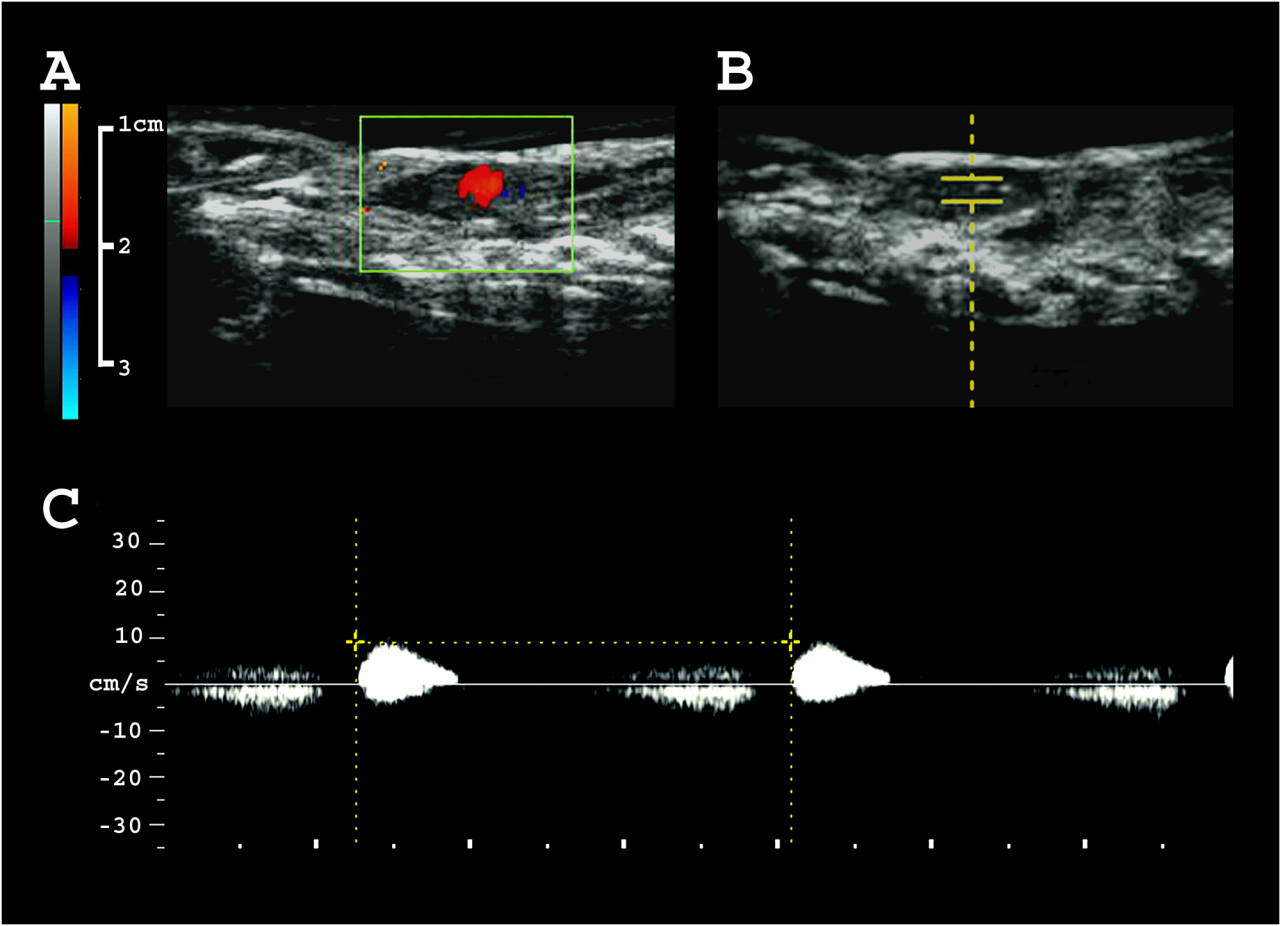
Measuring heart rate by ultrasonography. A 2D color Doppler image of the beating heart. B B-mode image indicating the localization of pulsed wave Doppler acquisition. C Pulsed wave Doppler of the beating heart.
